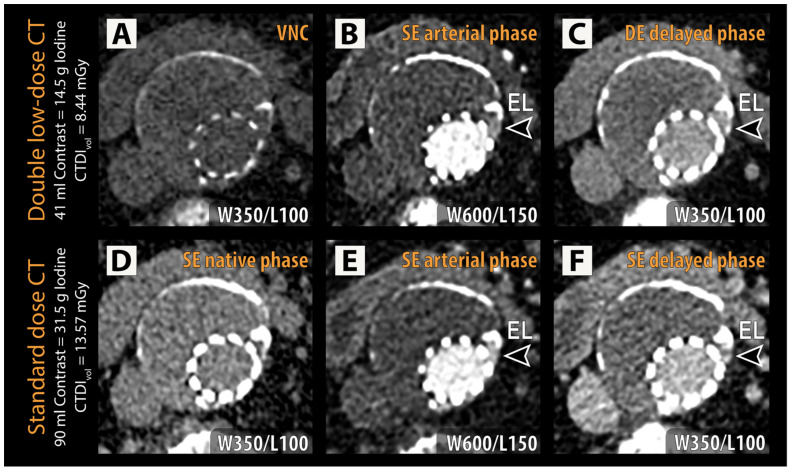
Representative examples of double low-dose CT (A–C) and reference standard CT (D–F) are shown with identical window/level (W/L) settings for each phase. An endoleak (EL, arrowheads) can be identified in the left lateral aspect of the excluded aneurysm sac adjacent to the stent struts in both the arterial phase (B,E) and delayed phase (C,F) in the dual low-dose CT and standard-dose CT, respectively. CTDI_vol_ = volumetric CT dose index; VNC = virtual non-contrast.
